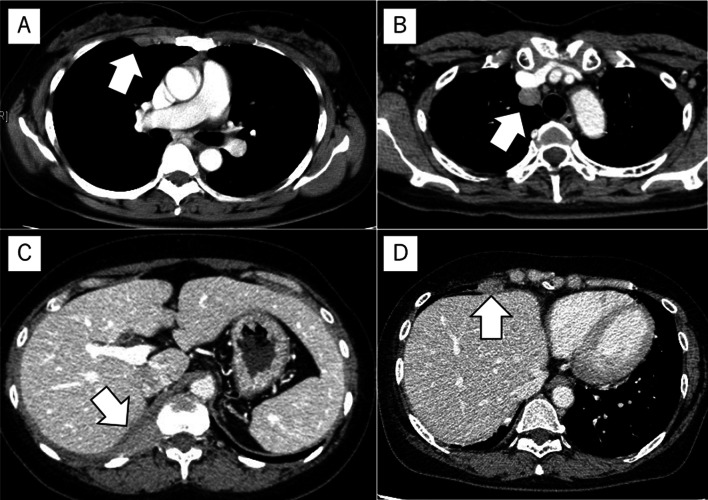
Chest computed tomography (CT) imaging of the disseminated pleural nodules seen in the postoperative recurrence of thymic carcinoma (arrows). A CT taken during the 3rd postoperative year. A nodule is noted on the anterior chest wall. B, C CT taken during the 10th postoperative year. A nodule is observed right behind the right brachiocephalic vein (B) and at the crus of the diaphragm (C). D CT taken during the 16th postoperative year. A nodule is shown between the anterior chest wall and the diaphragm
